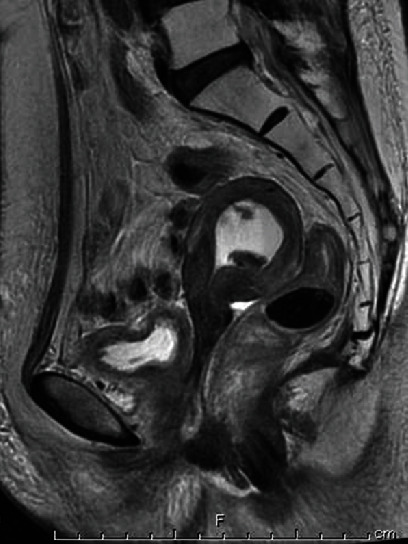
Magnetic resonance imaging (MRI) findings of endometrial tumors arising after pelvic radiation therapy. T2-weighted MRI showing markedly high-intensity liquid and two tumors in the uterine cavity (arrows).
